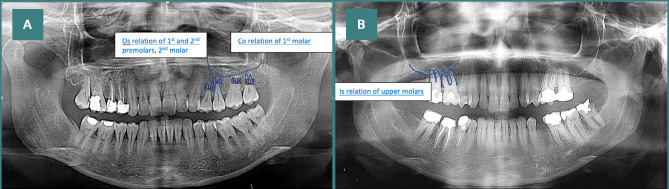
Examples of (A) Co and Os and (B) Is relations of teeth to the MS showing in panoramic radiographs
